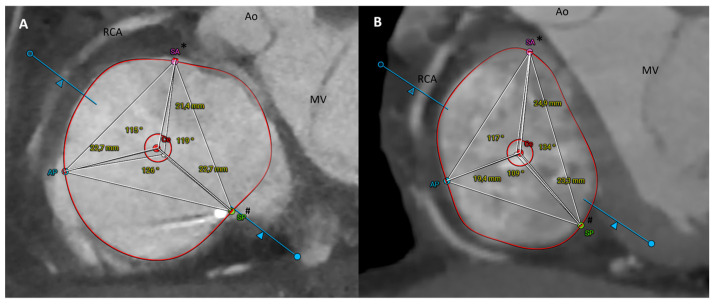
CT scan analysis using 3mensio Structural Heart 10.3 (Pie Medical Imaging, Maastricht, The Netherlands) in a patient with severe tricuspid regurgitation (A) and control (B). The different geometrical shape of the tricuspid annulus is appreciable: quite circular in (A) and more oval in (B). AP: antero-posterior commissure; Ce: centroid; SA: septal-anterior commissure SP: septal-posterior commissure. *SA corresponds to AS antero-septal commissure in the main text and tables; ^#^SP corresponds to PS postero-septal commissure in the main text and tables.
